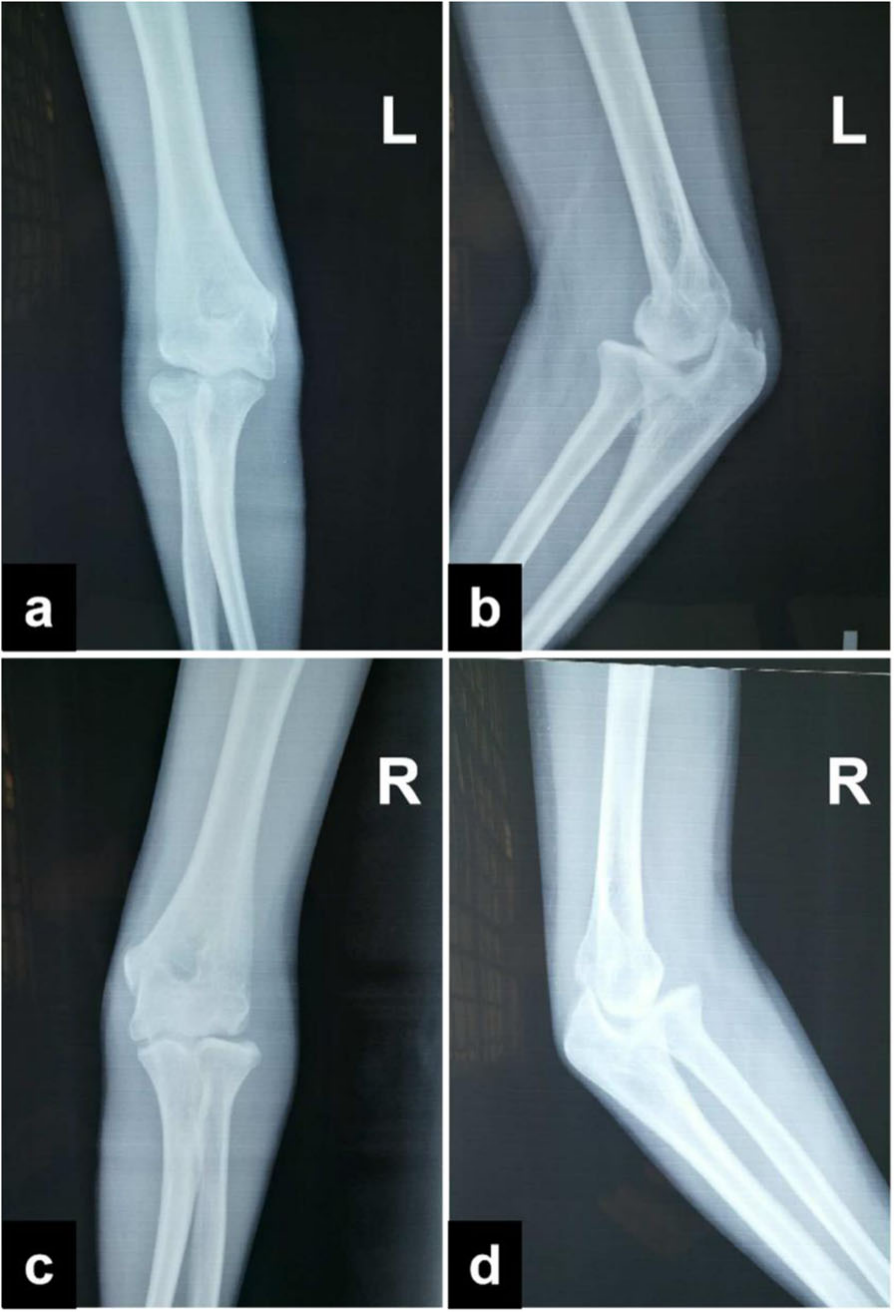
X-ray showing no calcific densities in bilateral elbow after operation
